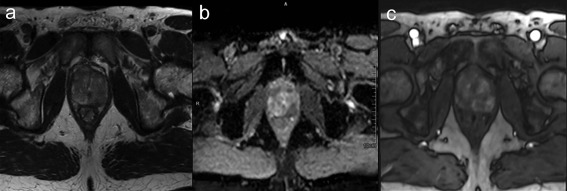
Multiparametric prostate MRI demonstrates a left mid‐gland PI‐RADS 5 peripheral zone lesion extending beyond the prostate (a: T_2_‐weighted, b: diffusion‐weighted apparent diffusion coefficient map, c: arterial phase dynamic contrast‐enhanced T_1_‐weighted image).
